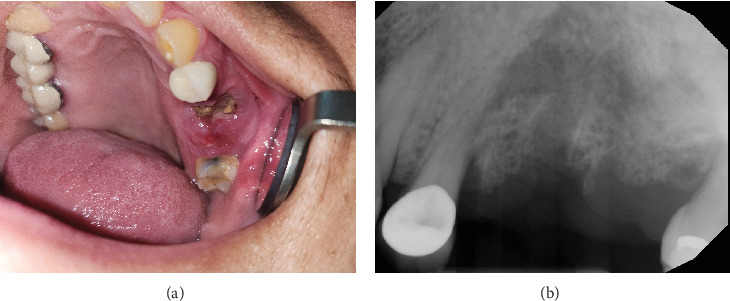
A 59-year-old female with medication-related osteonecrosis of the jaw. (a) The exposed necrotic bone of the left maxillary alveolar ridge measuring 15 mm × 10 mm in size and (b) periapical radiograph showing nonhealing extraction sites with mixed radiopaque and radiolucent areas.
